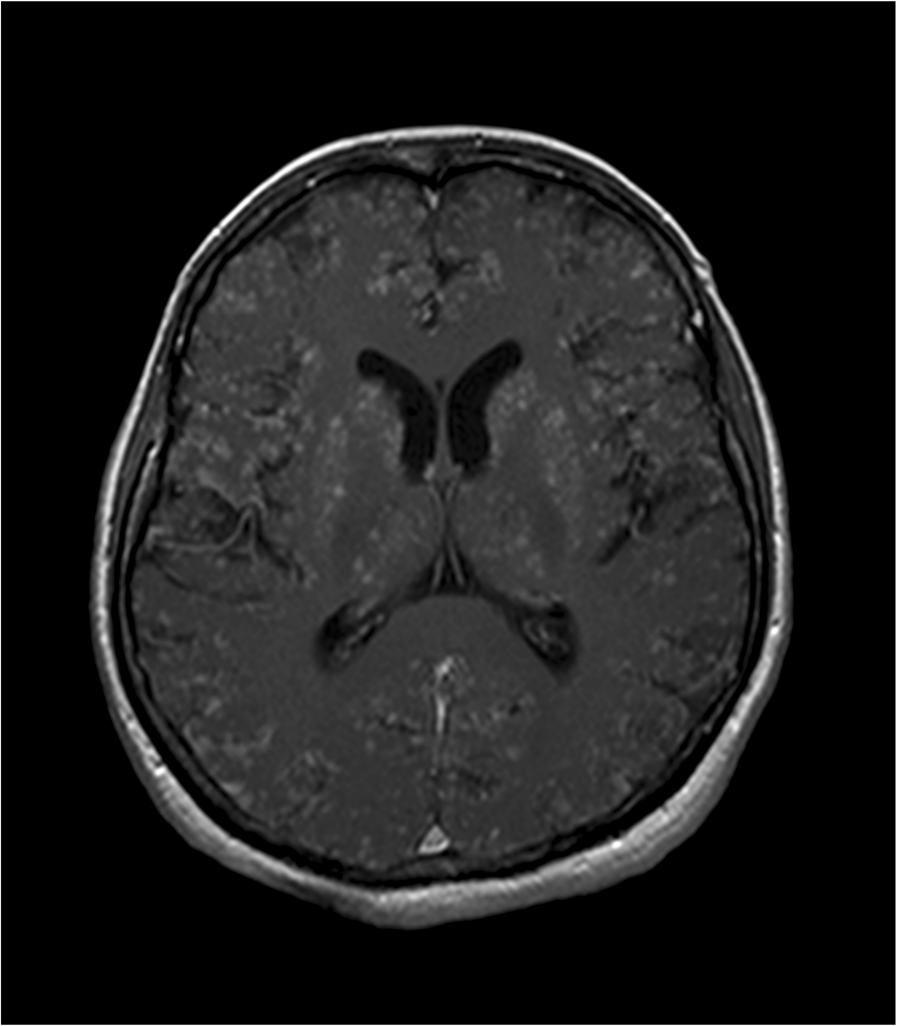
T1-weighted sequence of MRI. The axial T1-weighted sequence after intravenous administration of a Gadolinium-based contrast medium demonstrates a military pattern of innumerable small contrast-enhancing lesions with a predilection of the grey matter including the cerebral cortex, basal ganglia and thalami
